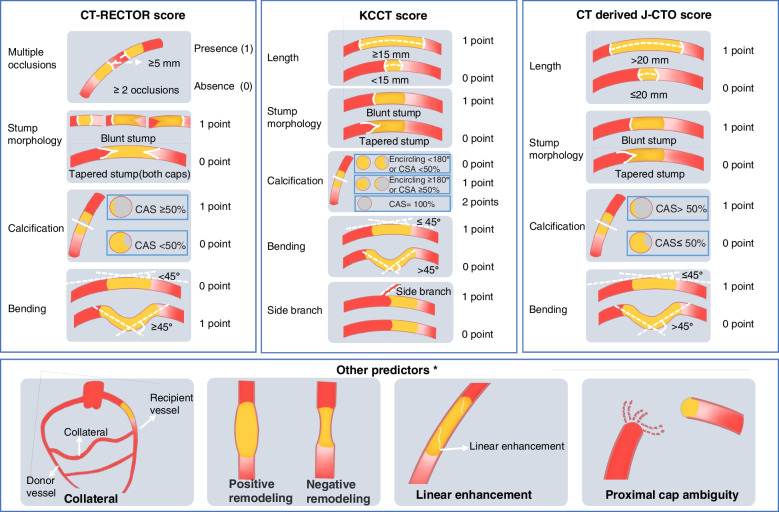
Scoring systems on CCTA and other predictors. CT-RECTOR, Computed Tomography Registry of Chronic Total Occlusion Revascularization; KCCT, Korean Multicenter CTO CT Registry; J-CTO, Multicenter CTO Registry of Japan; CAS, cross-sectional area. *Predictors not mentioned in the score systems based on CT
